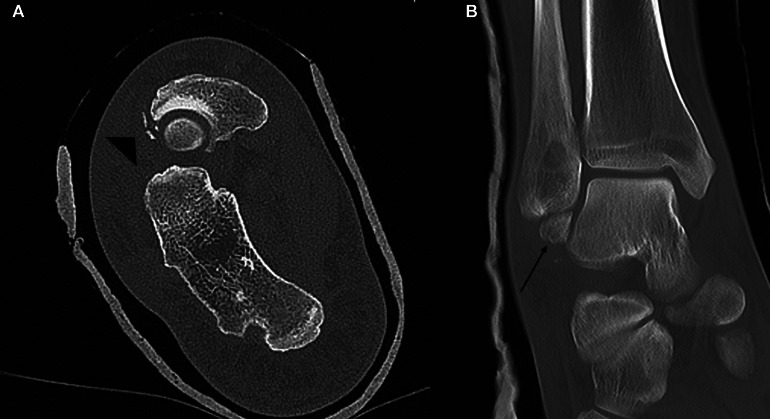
Postreduction axial (A) and coronal (B) CT slices of the right ankle. There are small avulsed bone fragments from the lateral aspect of the navicular (arrowhead). There is a well-corticated bone fragment inferior to the lateral malleolus in keeping with an old fracture (arrow).
